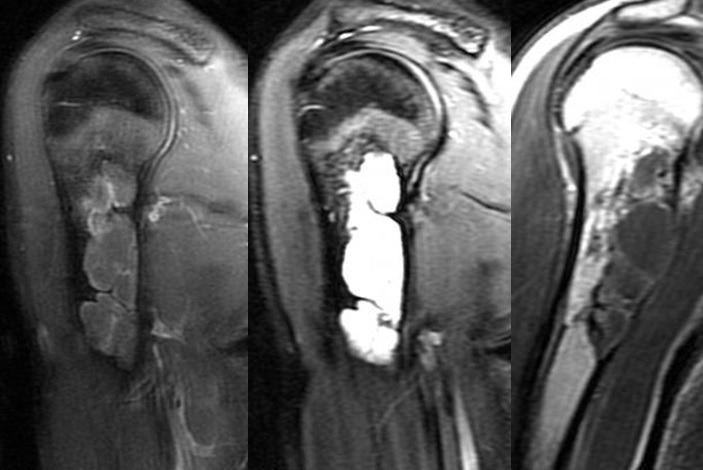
MRI imaging of the recurrence
